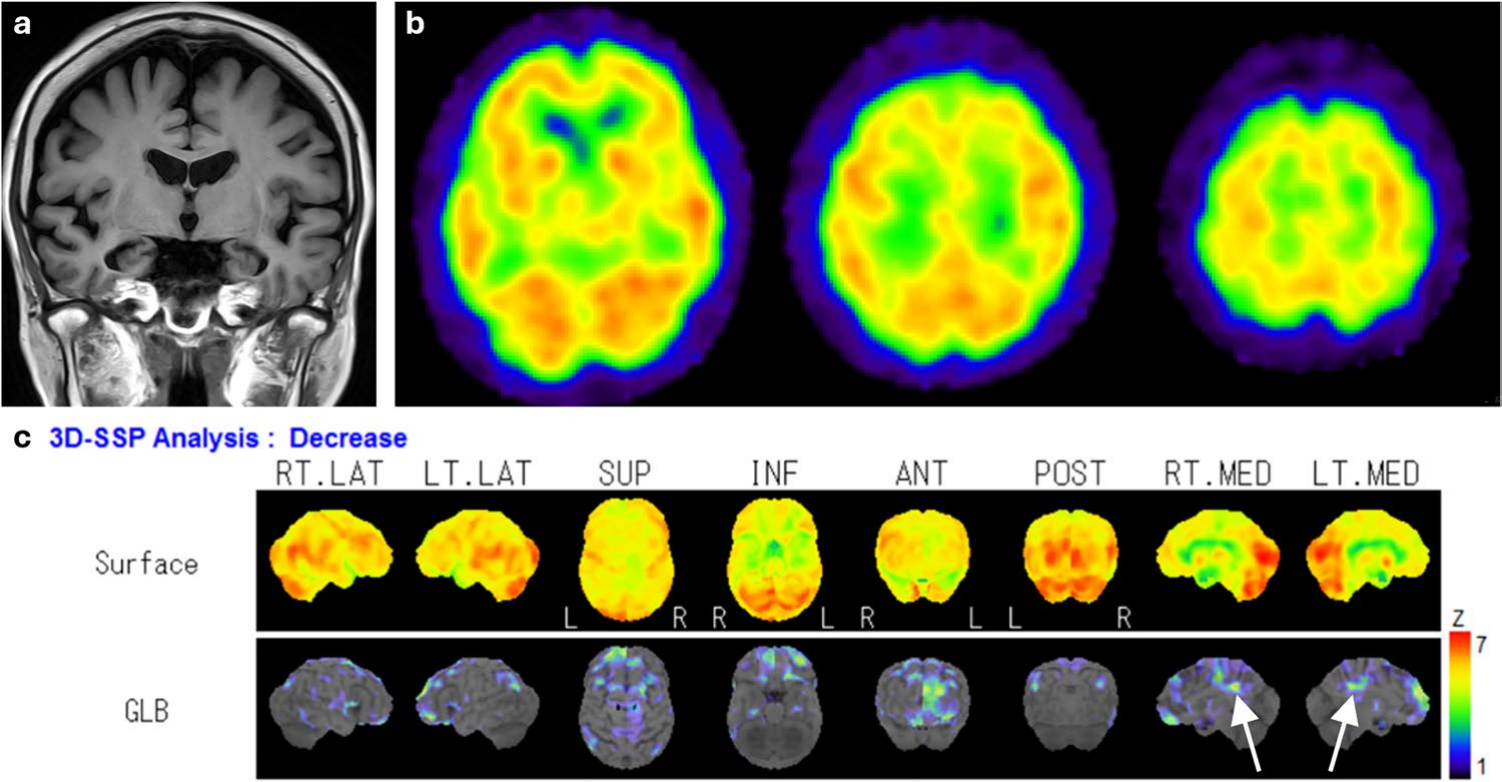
A 76-year-old female with MCI. Elevated CSF pTau and decreased CSF Aβ-42 levels, along with a positive ^18^F-Florbetapir PET scan (not shown), were indicative of AD. FLAIR coronal image revealed bilateral medial temporal lobe atrophy. IMP brain perfusion images exhibited no evident hypoperfusion. However, 3D-SSP analysis detected hypoperfusion in the posterior cingulate gyrus (arrows), consistent with AD
